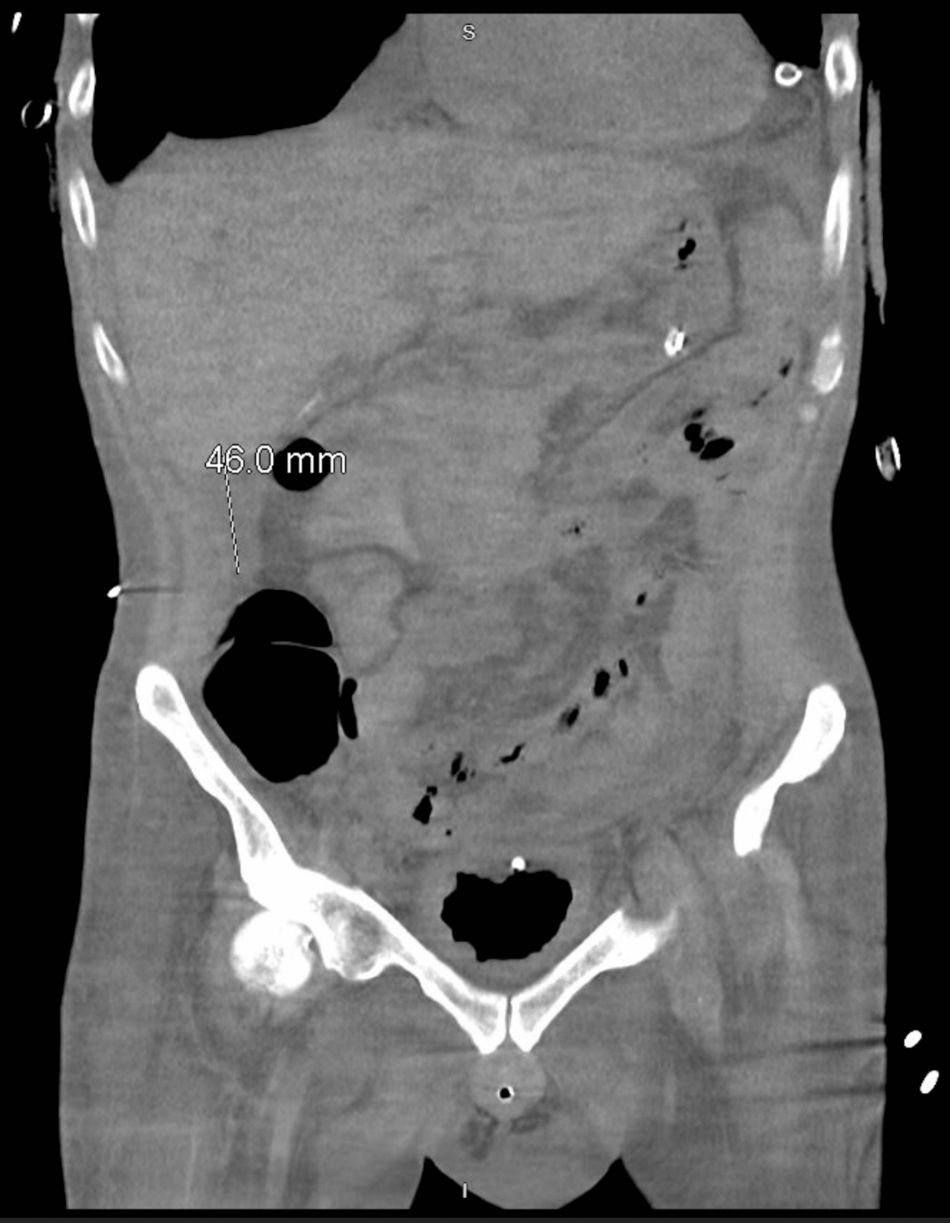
A CT scan of the abdomen demonstrates fluid collection below the liver which spans 46 mm.
